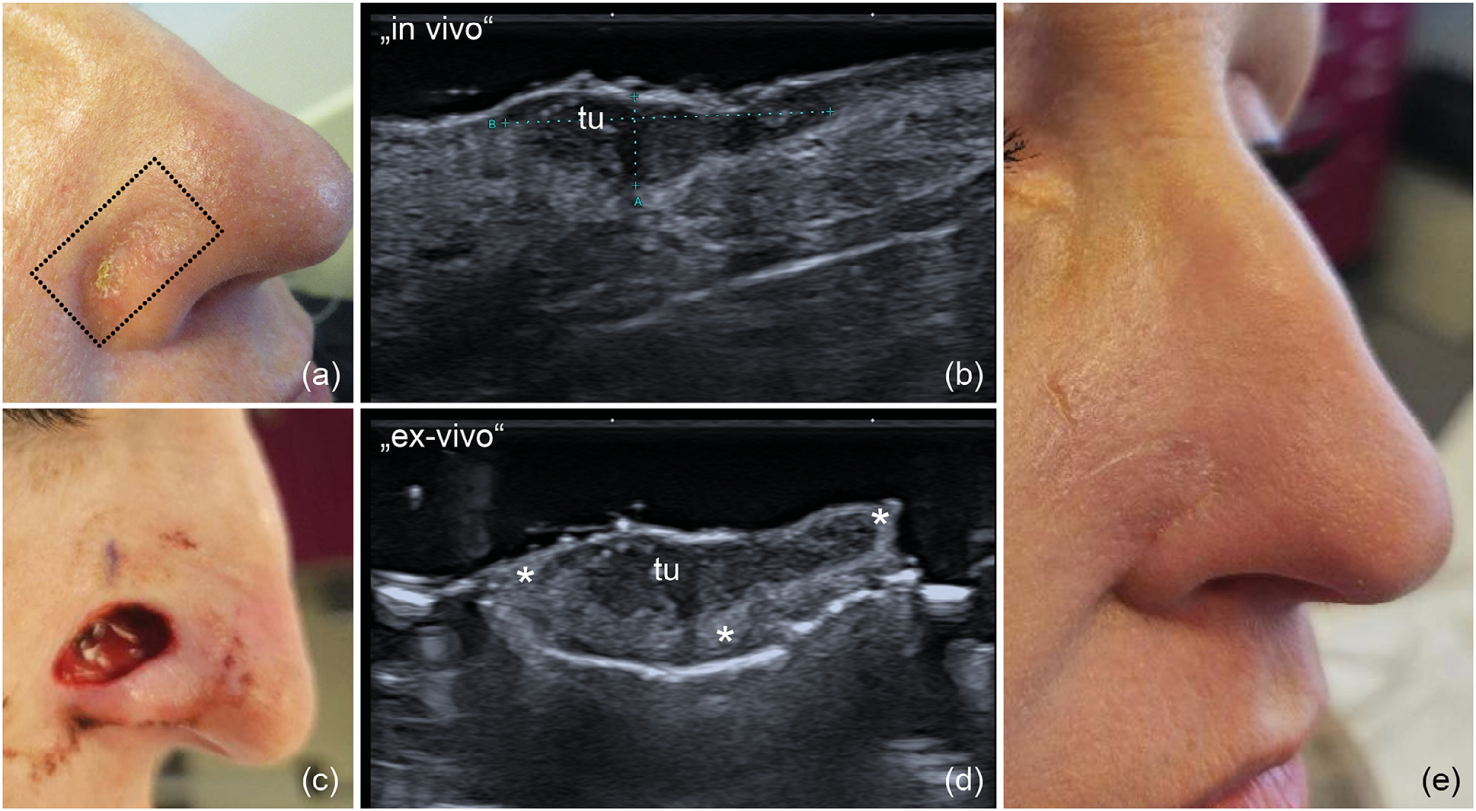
(a) Clinical aspect of an infiltrative BCC at the right nasal ala; the rectangle indicates the placement of the sonographic probe; (b) In vivo sonography displaying a hypoechoic lesion with hyperechoic spots, located in the dermis and extending into the subcutaneous tissue; (c) *ex vivo* sonography of the resected tumor, showing safety margins in depth and on both sides, marked by “*”; (d) clinical aspect of the surgical defect after tumor resection; (e) surgical site four weeks after defect reconstruction with a rhombic flap.
